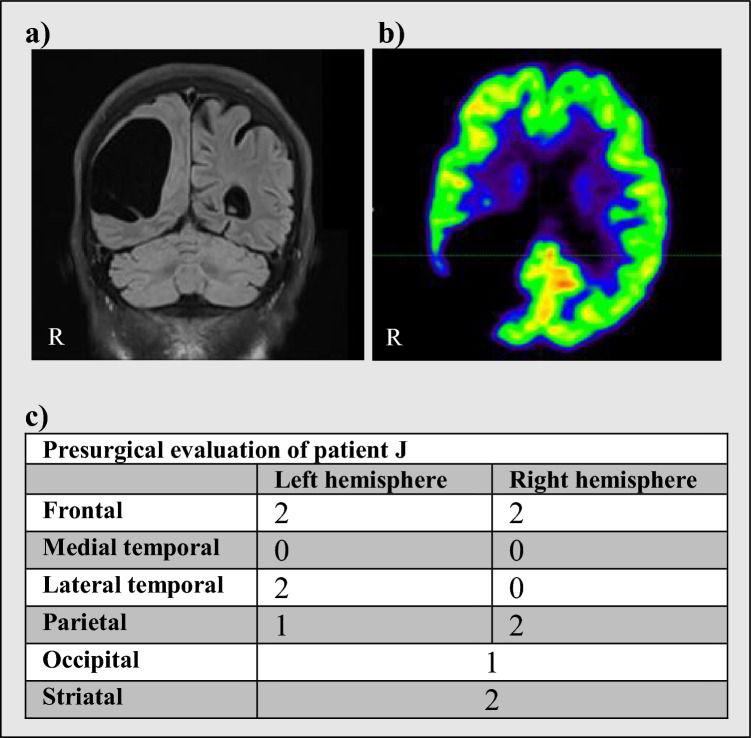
Patient J. Patient J was a 73-year-old female with complaints of memory impairment, problems with word retrieval, troubled spatial orientation, depressive symptoms and anxiety. A right parietal arachnoid cyst had previously been detected and dismissed as asymptomatic. New MR images revealed progression of cyst volume from 61 cm^3^ to 104 cm.^3^ and emergence of a mass effect with compression of surrounding sulci and gyri. The right thalamus was displaced. An FDG scan revealed an increased uptake in the right hemisphere and reduced uptake in the left cerebellar hemisphere. Neuropsychological examination revealed dysfunction of several cognitive domains. The patient had surgery which was followed by normalization of parenchyma on MR but the patient did not experience subjective alleviation of symptoms. Neither neuropsychological examination nor FDG scanning was repeated. The patient was not diagnosed with dementia. a) MR. b) FDG-PET. c) Neuropsychological scores (see 2.2 Neuropsychological profiles for explanation)
